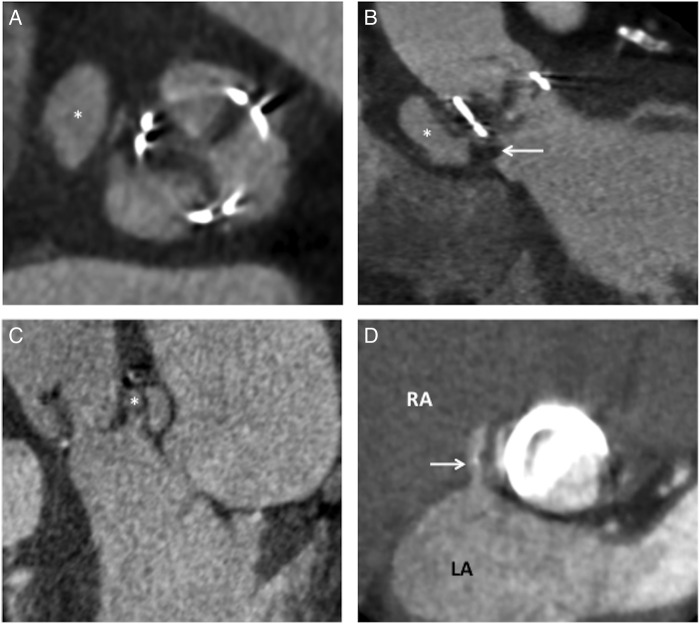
Prosthetic valve infective endocarditis. (A) Complications of prosthetic valve infective endocarditis include the development of perivalvular pseudoaneurysm formation (white asterix). (B) Saccular pseudoaneurysms (white asterix) occur in regions where vegetations (white arrow) erode through the annulus with a loss of sewing ring integrity. (C) Bacterial spread into the aortic root results in abscess formation (white asterix). (D) The anatomical location of erosive shunts (white arrow) and paravalvular leaks due to suture dehiscence can be readily identified using cardiac CT. LA, left atrium; RA, right atrium.
